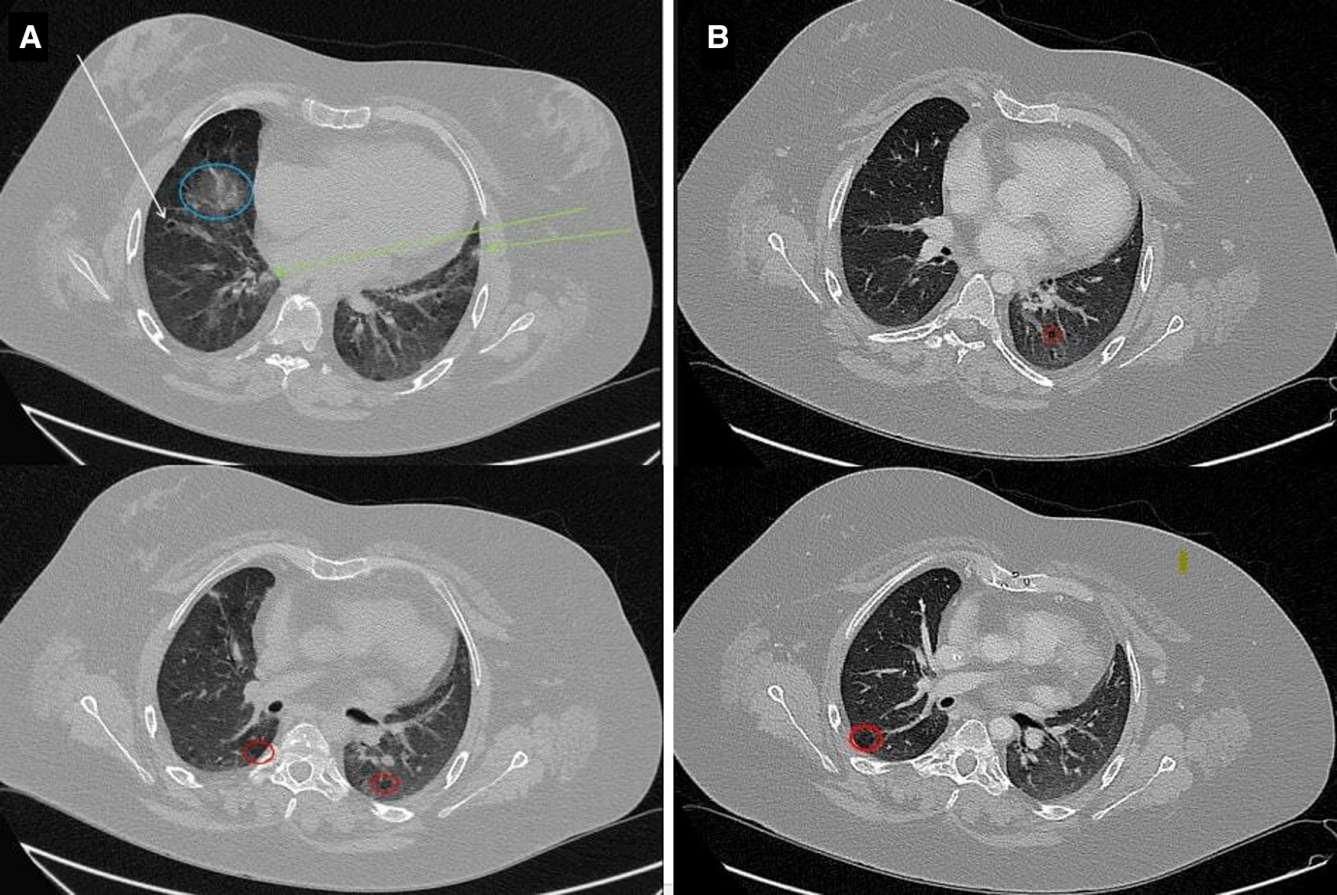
Chest HRCT. (A) Images show interstitial lung disease and chronic inflammation. Note the numerous findings, including ground-glass opacity (blue circle), bronchiectasis (white arrow), nodules with blurred edges (green arrows), and regions of air trapping (red circles). (B) Radiological disease improvement with persistence of air-trapping.
